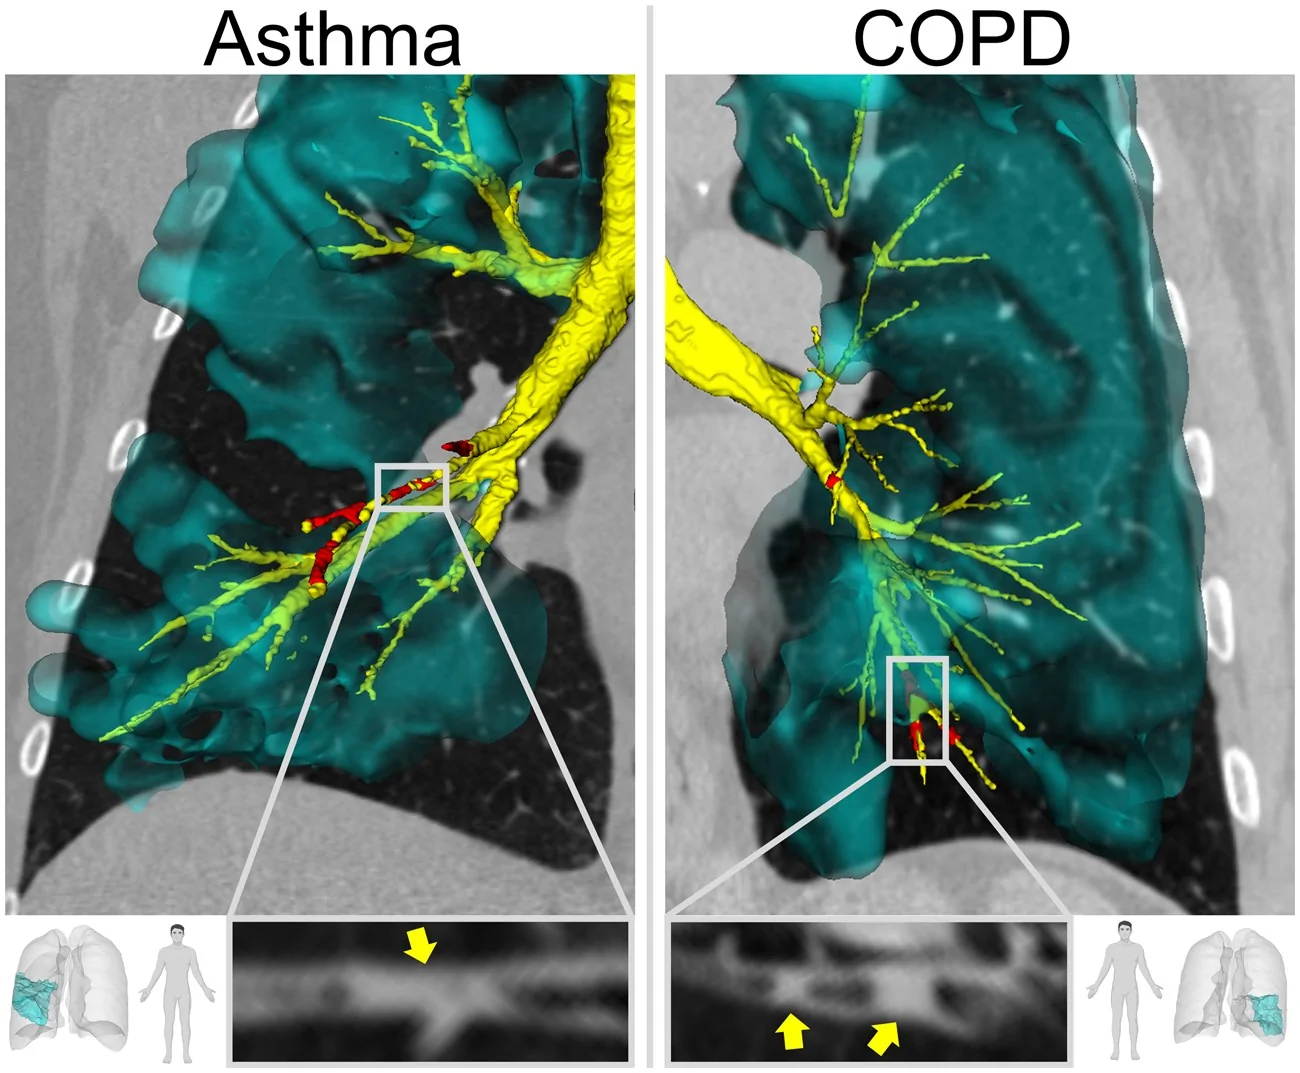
Mucus plug visualization within 3D airway tree. Coronal reconstruction of computed tomography with 3D airway tree (yellow) and center coronal slice ^129^Xe MRI ventilation (cyan) with mucus plug (red) enlarged in inset. Left: Front view of a 59-year-old woman with severe asthma; mucus plug score of 38, mucus plug segment score of 10, prebronchodilator FEV_1_% predicted = 73%, SGRQ score = 75, and VDP = 41%, with a visually obvious ventilation defect in the right middle lobe and “stringy” mucus plug in the lateral segmental bronchus (yellow arrow). Right: Front view of a 63-year-old man with GOLD stage 3 COPD, mucus plug score of 20, mucus plug segment score of 8, prebronchodilator FEV_1_% predicted = 38%, SGRQ score = 77, and VDP = 23%, with a visually obvious ventilation defect in the left lower lobe and “stubby” mucus plug in the lateral segmental bronchus (yellow arrows). Image courtesy of the research team of Grace Parraga, PhD. Abbreviations: COPD, chronic obstructive pulmonary disease; GOLD, Global Initiative for Chronic Obstructive Lung Disease; MRI, magnetic resonance imaging; SGRQ, St. George’s Respiratory Questionnaire; VDP, ventilation defect percentage.
